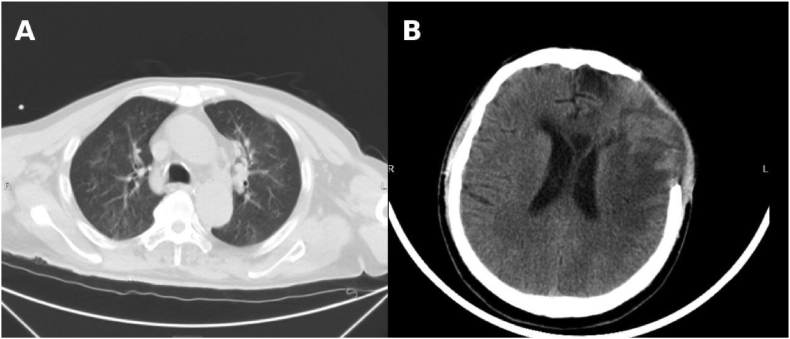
Follow-up imaging after surgical intervention and voriconazole therapy. (A) Follow-up chest CT after voriconazole therapy showing marked improvement of pulmonary lesions. (B) Postoperative brain CT demonstrating stable postoperative changes without evidence of new intracranial lesions.
